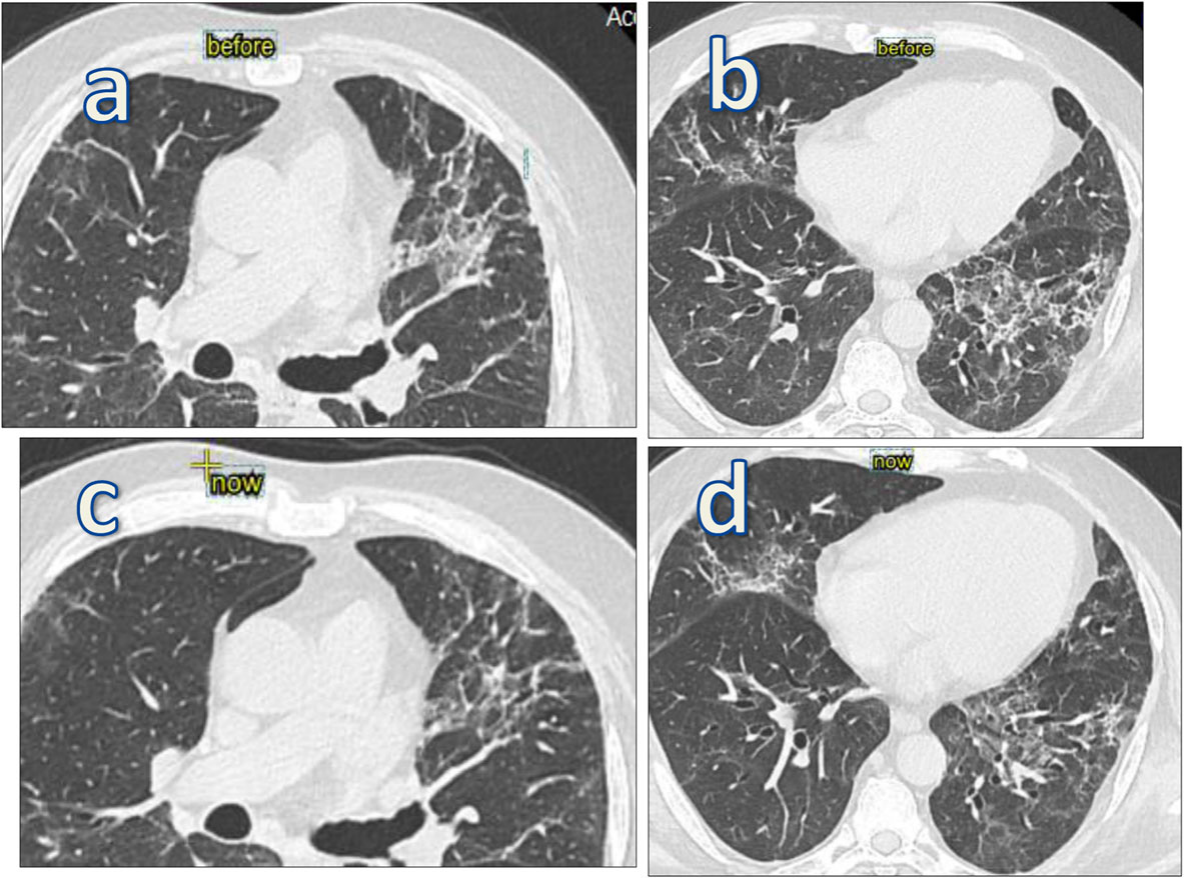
A 64-year-old male patient presented with fever, severe dysnea, and cough; first MSCT was done on the 12th day of manifestation showing classic CT finding of COVID-19, CT-SS 18/25, (bilateral peripheral subpleural ground glass opacifiection with peribronchial thickening and atelactatic bands) (a, b). Patient came on 12th week with persistent dysnea, axial cuts MSCT (c, d) was done showing regression regarding ground glass opacities, with persistent fibrotic changes (peribronchial fibrosis, archeticture distortion, and traction bronchectatic and bronchilectatic changes)
